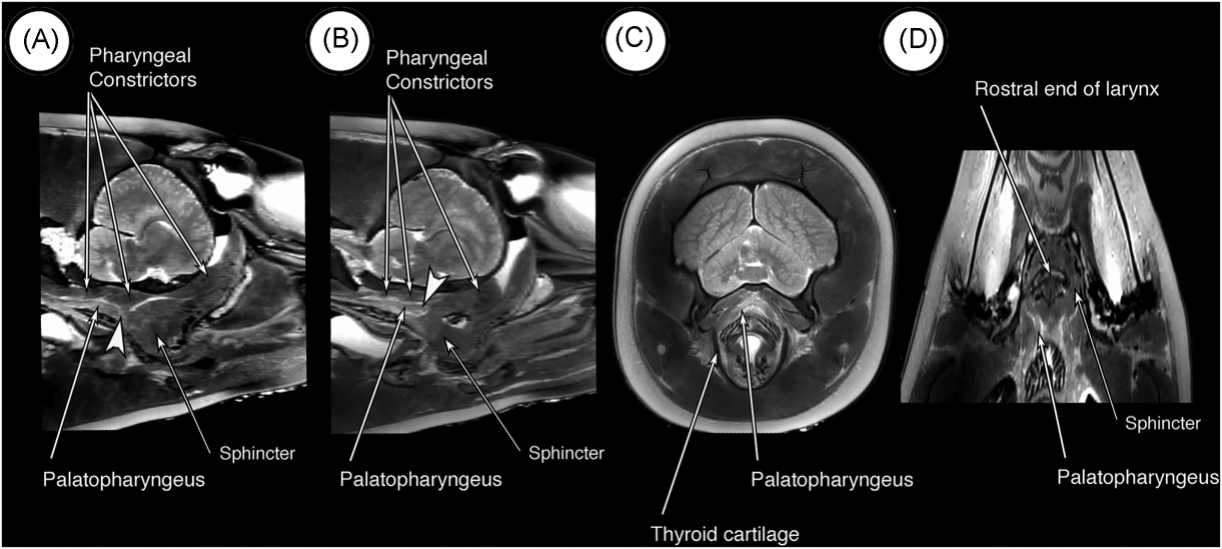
MRI scans in the sagittal (A, B), transverse (C), and dorsal (D) planes showing the palatopharyngeus muscles. (A) The palatopharyngeus muscle on each side is a longitudinal muscle deep to the pharyngeal constrictors. As the muscle inserts into the palatopharyngeal sphincter, it divides into two parts. Shown here is the more lateral part (arrowhead) that inserts ventrally into the sphincter. (B) Shown here is the more medial part of palatopharyngeus as it inserts dorsally into the sphincter. (C) In this transverse scan the muscle attaches to the thyroid cartilage. (D) In this scan in the dorsal plane, the palatopharyngeus muscles are shown inserting into the sphincter.
